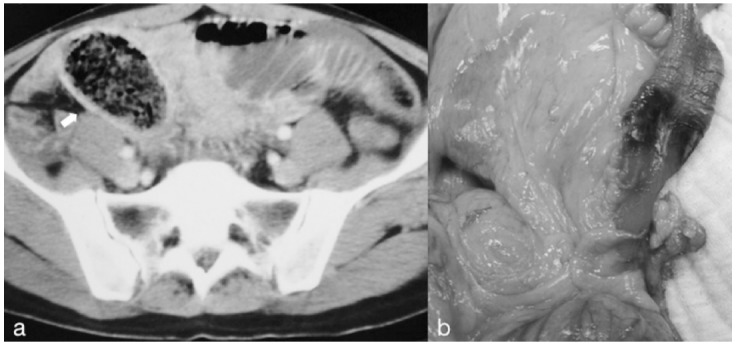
Perforation due to phytobezoar: intraluminal bezoar imaging and inflammation findings in surrounding tissues on CT (a), intraoperatif view; necrotic area and perforation at ileum depending on bezoar pressure (b)
